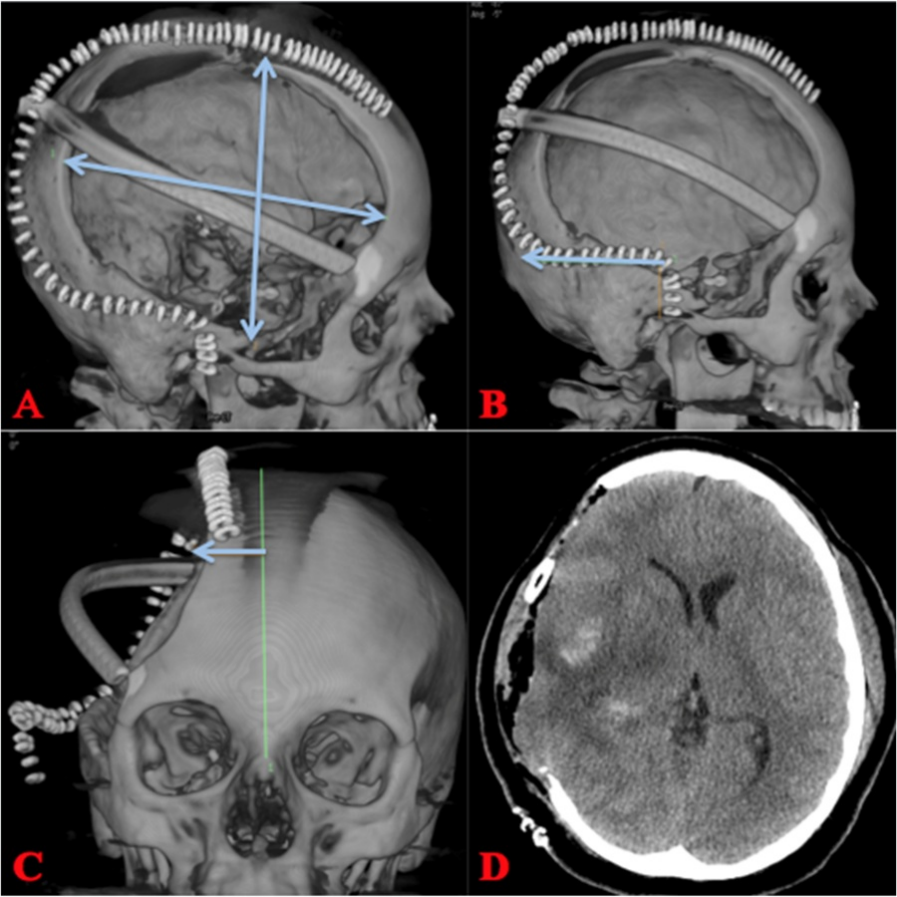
Postoperative Hemicraniectomy A, B) A large right hemicraniectomy with dimensions that should be 15 X 12 cm (AP & lateral). The incision should be carried posteriorly for 6 cm from the temporal root of zygoma. C) Incision should be carried to within a couple centimeters from midline to avoid the superior sagittal sinus. D) Postoperative CT showing craniectomy defect and improvement in mass effect. AP: anteroposterior; CT: computed tomography
